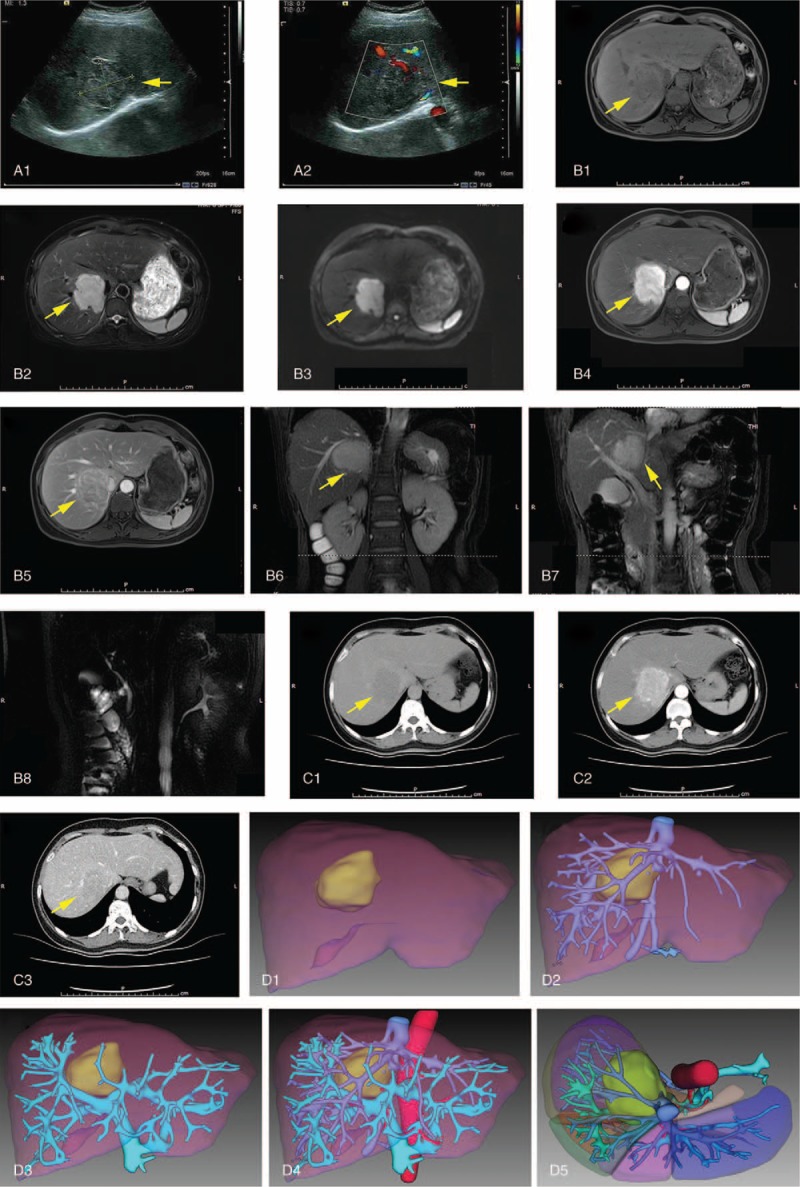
Preoperative radiological examination of the reported case. (A) Ultrasonography revealed a hypoechoic mass with its diameter of 5.7 × 4.9 cm (A1), color Doppler flow imaging indicated that the mass was next to the second porta of liver (A2). From (A) to (C), yellow arrows heads direct the liver lesion; (B) abdominal MRI scanning. T1-weighted magnetic resonance (MR) image showed a low signal intensity mass which located in the segment 7 and 8 and caudate process of the liver (B1), and T2-weighted MR image showed the lesion is of high signal intensity (B2); MR with diffusion-weighted images showed that the mass was hyperintensity with restricted diffusion (B3); MR with perfusion-weighted imaging showed the mass was hyperenhanced in the arterial phase (B4) and de-enhanced in the portal phase (B5); coronal view of 2D-FIESTA sequence images showed the mass is close to the right hepatic vein (B6) and the right posterior branch of the portal vein (B7); and MR cholangiopancreatography showed no obvious abnormality in the bile duct (B8); (C) abdominal computed tomography (CT) scanning. Plain CT scan showed a hypoattenuating liver lesion (C1); arterial phase showed nodular enhancement of the lesion (C2); portal phase showed a low density nodule(C3); (D) 3D reconstruction of CT images. anterior view (D1-D4) and upper view (D5) of the reconstructed images of liver are showed. The liver lesion is labeled with light green, liver is labeled with auburn, abdominal aorta, and hepatic artery are labeled with red, portal vein and its branches are labeled with cyan, and hepatic vein and inferior vena cava are labeled with blue.
